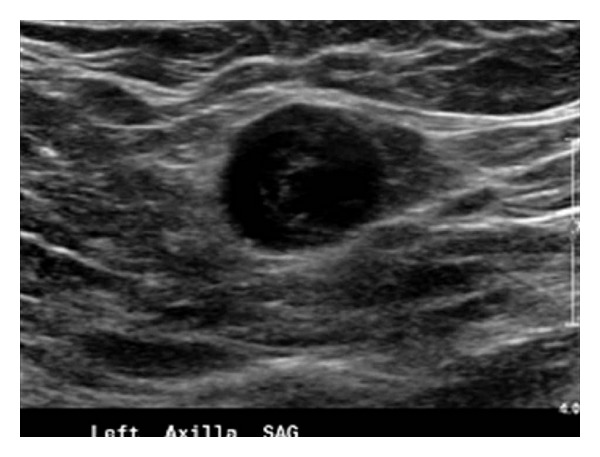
Ultrasound image at the time of core needle biopsy shows a well-circumscribed, 1.7 × 1.5 × 1.4 cm mass in the left axilla.
